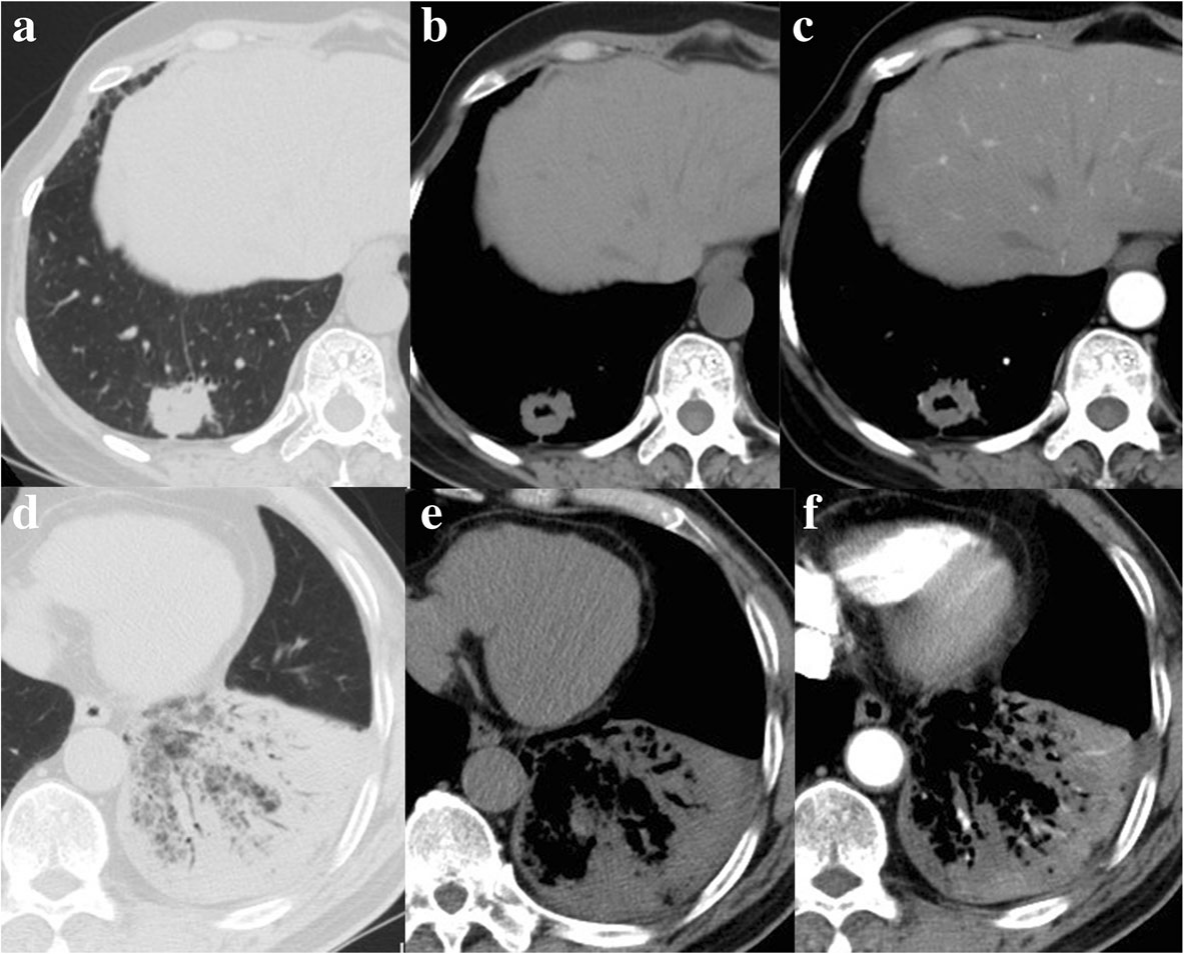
a-c A53-year-old female who presented solitary-type IMA on plain and contrast-enhanced computed tomography scans. d-f A73-year-old male who presented pneumonic-type IMA on plain and contrast-enhanced computed tomography scans
